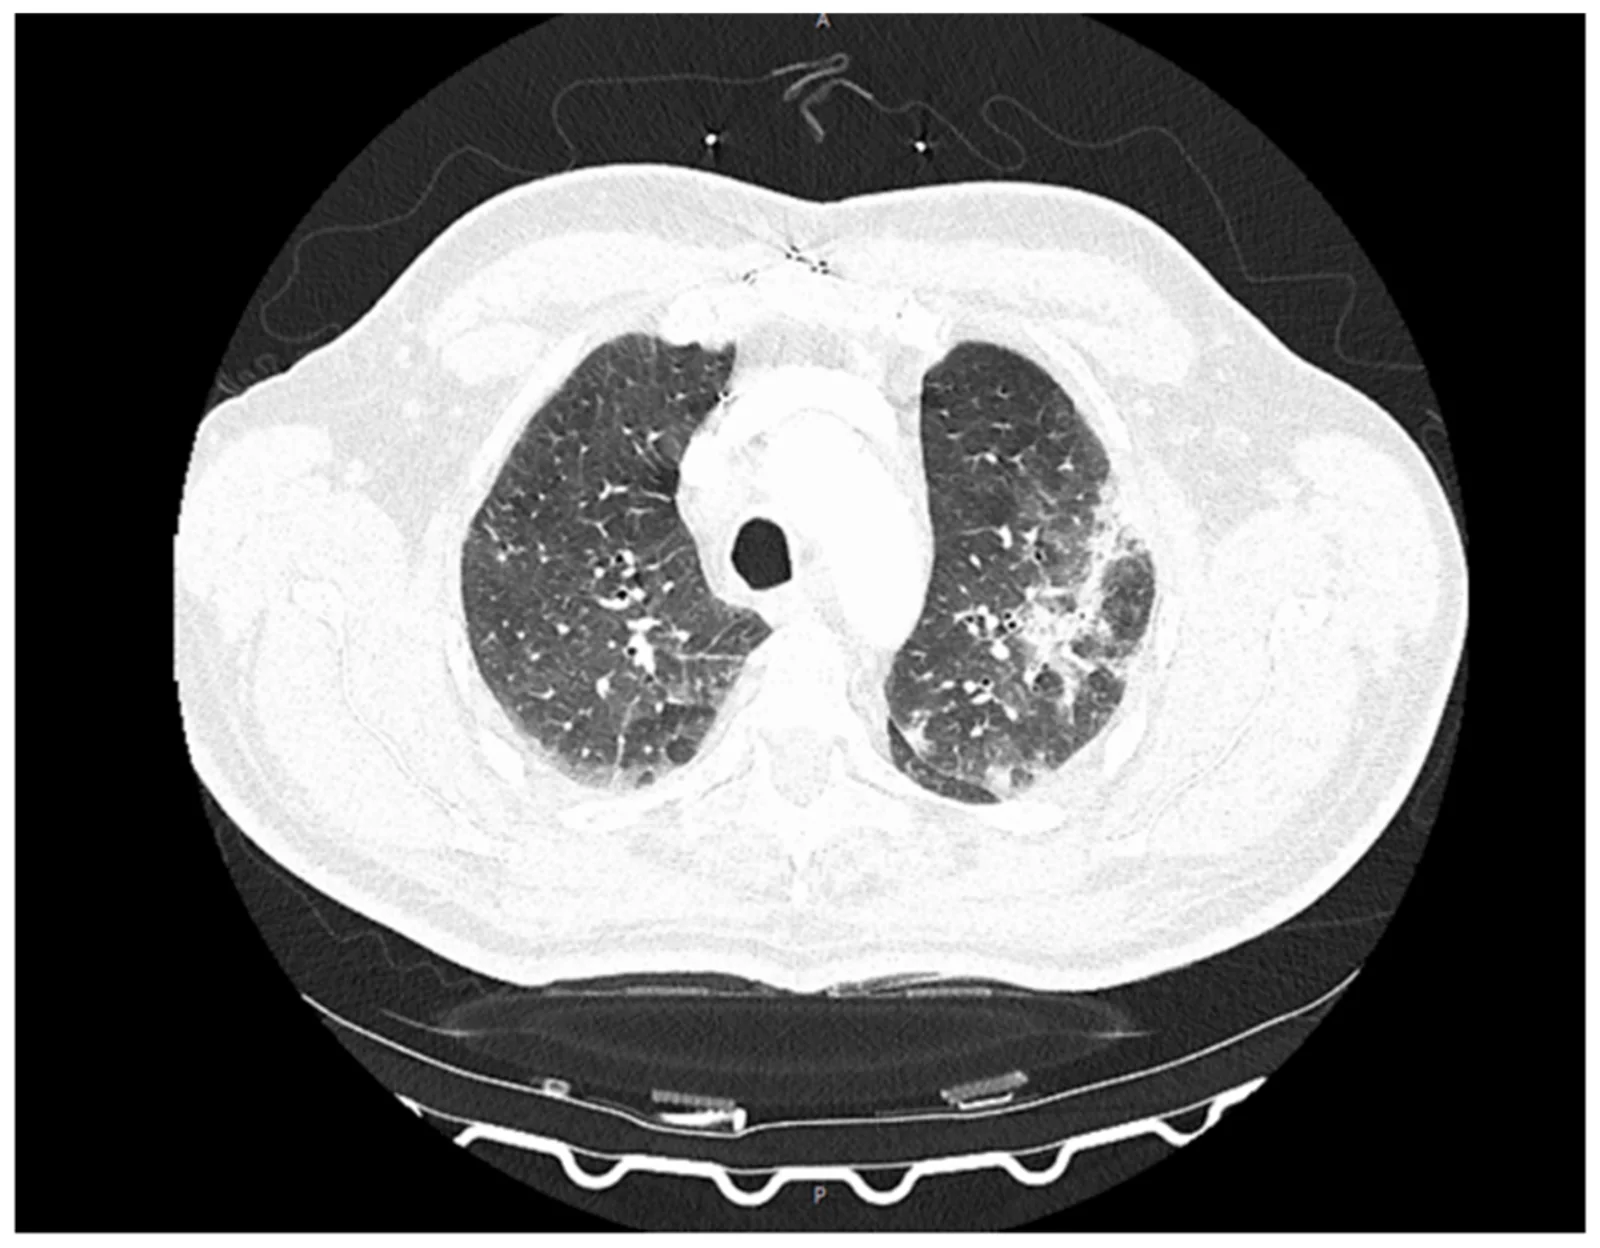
Serial chest CT scans demonstrating marked radiological improvement following combined therapeutic management with levofloxacin, systemic corticosteroids, HFNC, NIV, and awake APP.
